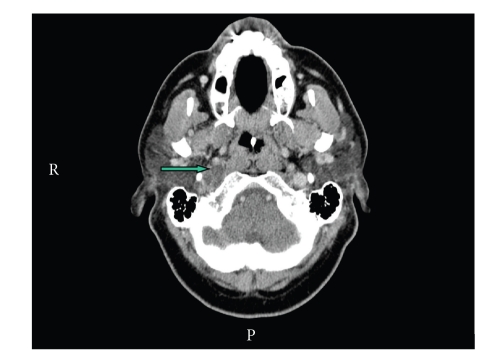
CT neck with contrast showing a low attenuating collection within the right carotid space, which demonstrates peripheral ring enhancement (block arrow). The appearances are suggestive of an abscess. No intracranial abnormality was identified.
